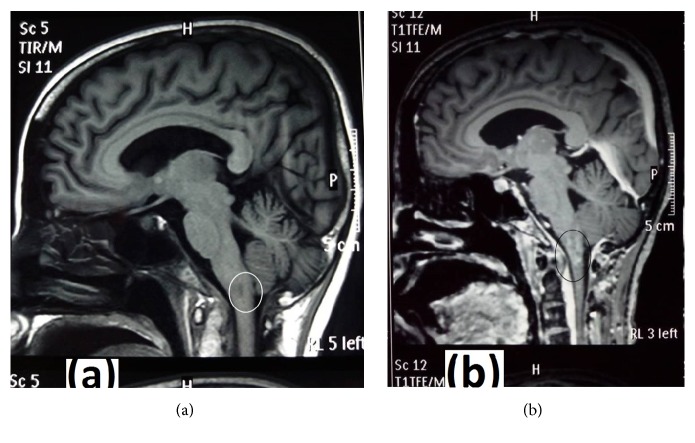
T1-weighted MRI of brainstem involving medulla oblongata demonstrating an infection: (a) without contrast; (b) postcontrast.
